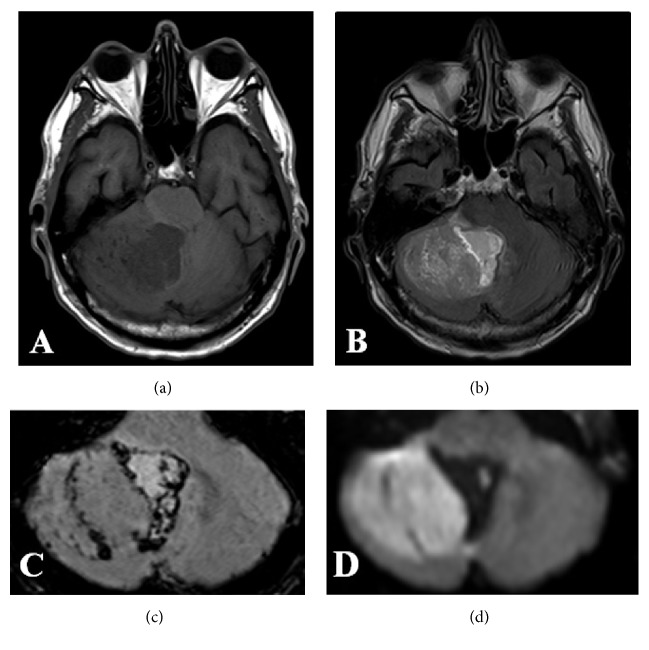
Transversal T1w image at 1.5 Tesla (a) shows a large hypointense tumor of the right cerebellar hemisphere with a hyperintense cyst on FLAIR images (b), which fills most of the fourth ventricle and causes obstructive hydrocephalus (not shown). Susceptibility weighted images (c) reveal hemosiderin deposits in the caudal tumor parts. Because of its dense cellularity, this tumor shows moderate restriction on diffusion weighted images (d).
